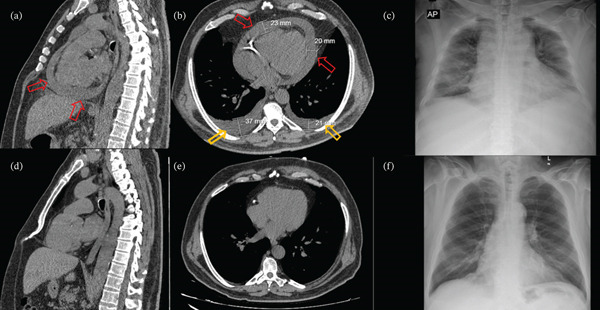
Computed thorax tomography imaging: Hospital admission (a–b), 1 year before (d–e). (a) Pericardial fluid accumulation indicated with red arrows in the sagittal section. (b) Pericardial fluid (red arrows) and pleural effusion (yellow arrow) shown in the transverse section. (c) Posteroanterior chest radiography with cardiomegaly and interstitial edema at hospital admission. (d–e) No pericardial or pleural effusion in previous computed thorax tomography. (f) Normal chest radiography at hospital discharge.
